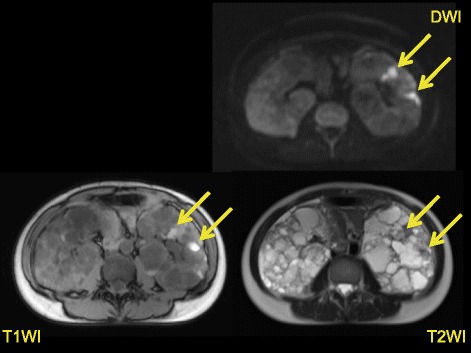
MRI findings (T1WI, T2WI, and DWI) in a control. The renal cysts show a higher intensity on DWI and T1WI than normal cysts, while having a lower intensity on T2WI. These are small cysts with a diameter of less than 5 cm
